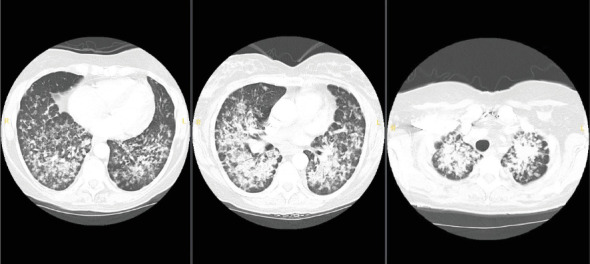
CT scans of the chest showing diffuse bilateral linear reticular and nodular infiltrates.
